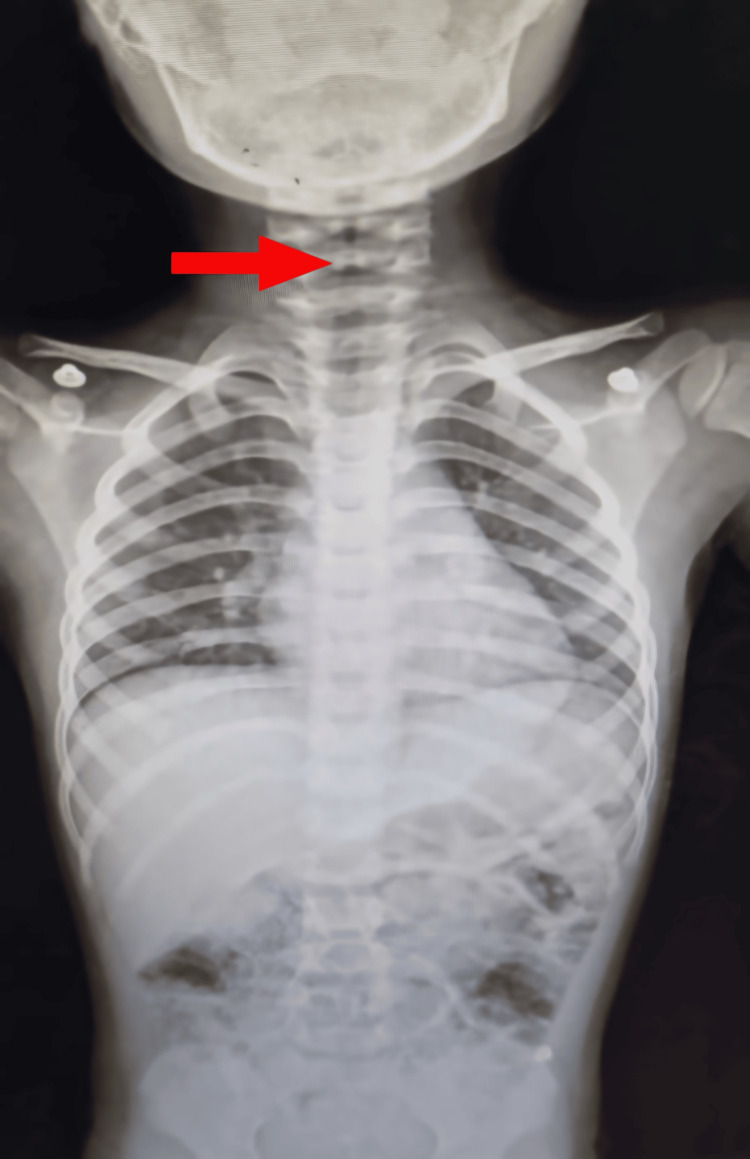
Posteroanterior chest radiograph demonstrating subglottic narrowing (steeple sign). The red arrow highlights the tapered narrowing of the upper trachea. This radiographic appearance mimics croup due to allergic subglottic edema.
